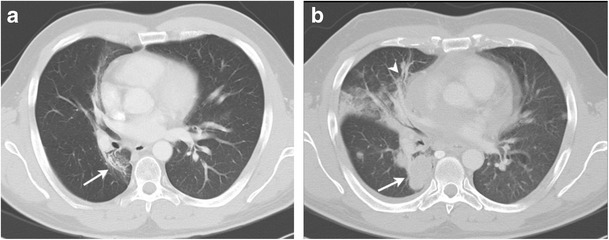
A 35-year-old male with a history of HL treated 3 years ago with chemotherapy and mediastinal radiation presenting with relapsed HL in the radiation field. a Axial CT image in lung window settings shows well-demarcated area of parenchymal distortion and traction bronchiectasis in a paramediastinal location in the right lower lobe (*arrow*) suggestive of post-radiation changes. b Axial CT image in lung window settings after 3 years shows the appearance of a lobulated mass within the radiation field with obliteration of the previously visualised air bronchogram (*arrow*). New appearance of ground-glass opacities with consolidation (*arrowhead*) is seen in the right middle lobe
